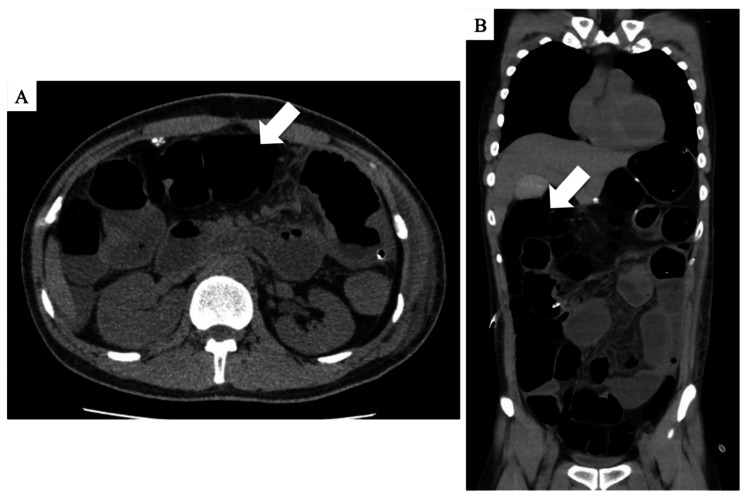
Abdominal CT scan obtained two days after the second surgery. Axial CT imaging showing persistent bowel distension (arrow), although no free intraperitoneal air is observed. These findings were interpreted as ongoing intestinal dilatation due to postoperative intestinal paralytic ileus. CT: computed tomography
